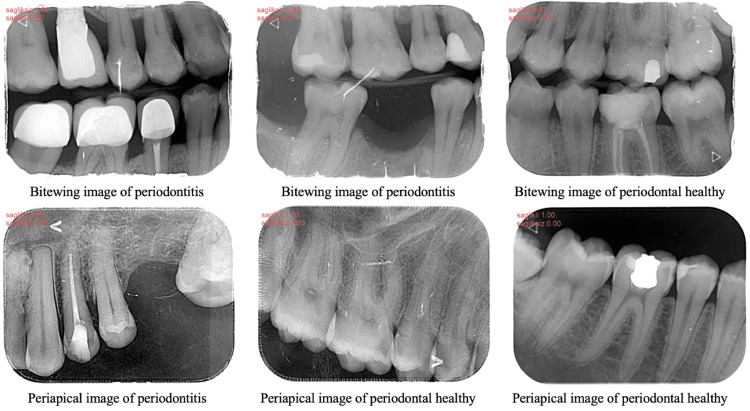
All images presented showing the output of the artificial intelligence (AI) algorithm developed for bitewing and periapical radiographs indicate that the system correctly diagnosed all images
